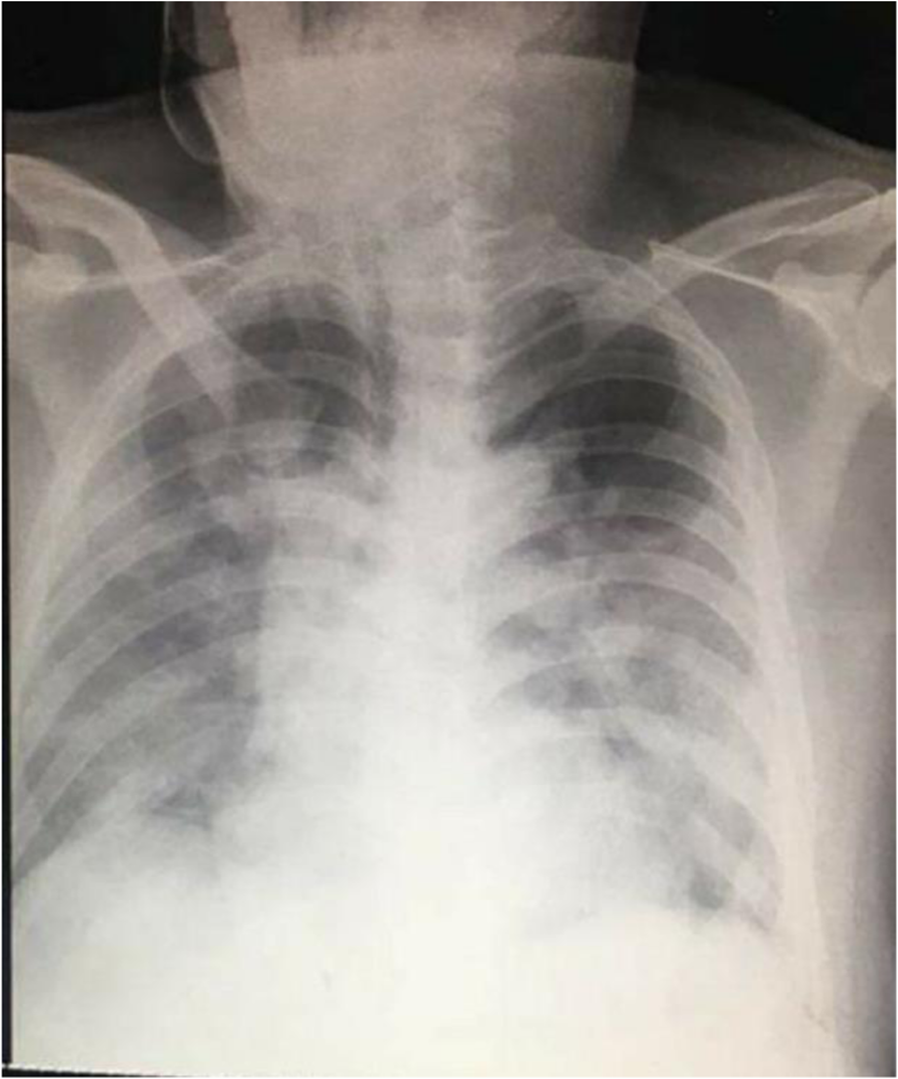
Posteroanterior chest radiograph of patient 2, 27 January 2020 (illness day 10). Hazy infiltrates in both lung fields consistent with pneumonia
